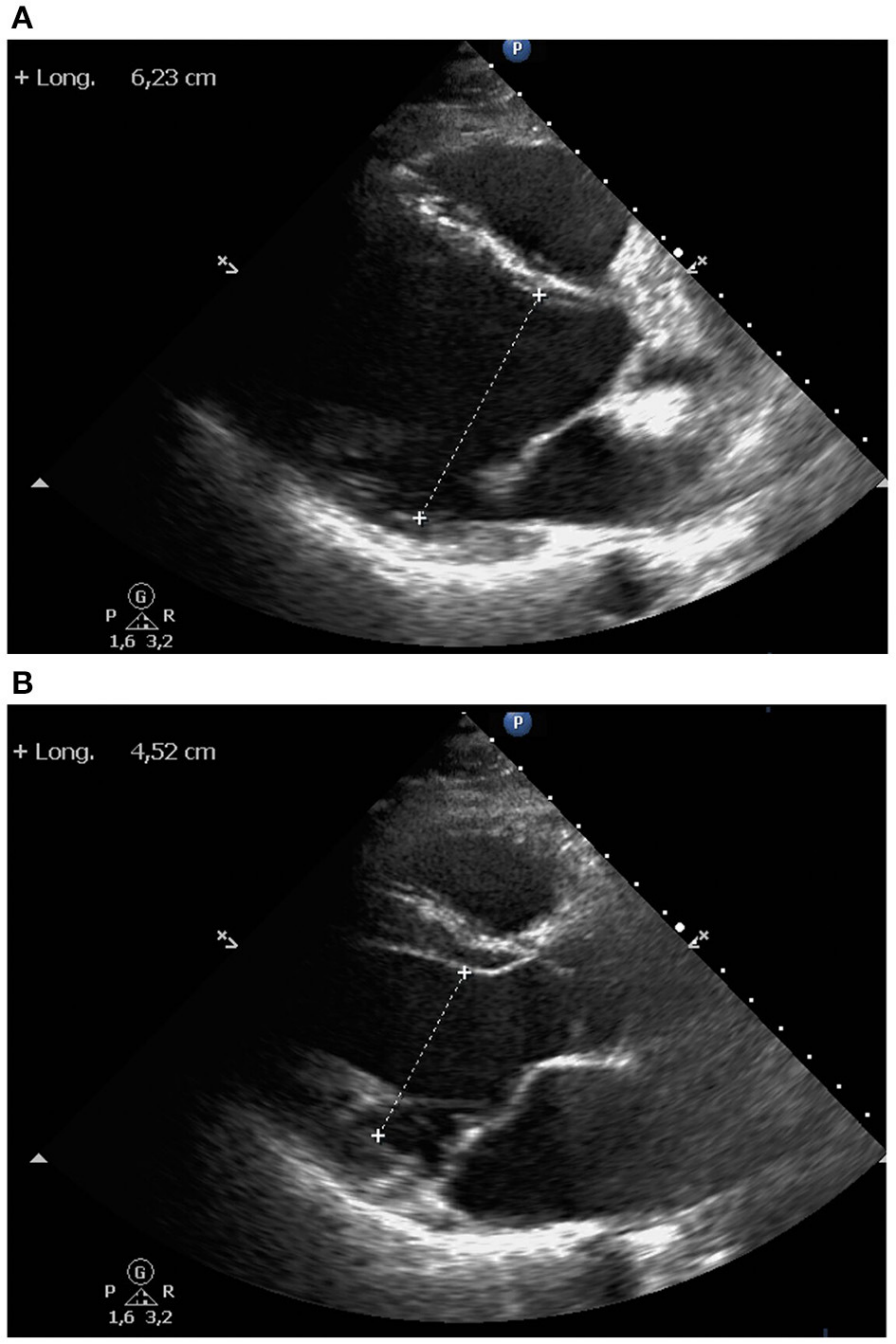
Echocardiography. Parasternal long axis view showed mild left ventricular enlargement, both left ventricular end-diastolic (A) and end-systolic (B) diameters. Right ventricle, ascending aorta and left atrium were of normal size. Interventricular septum and posterior left ventricular wall had normal thickness. No pericardial effusion was observed.
